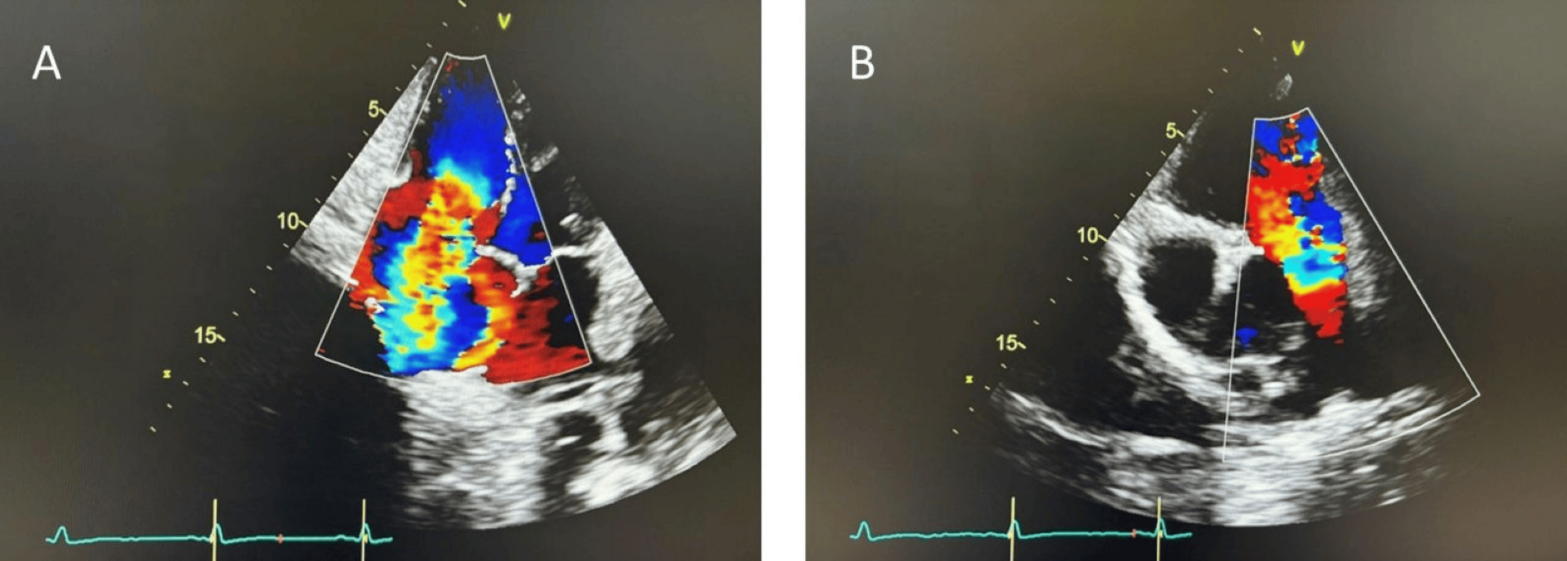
Transthoracic Echocardiography A) Apical four-chamber view showing severe free flow tricuspid regurgitation. B) Parasternal short-axis view showing moderate pulmonary regurgitation.
